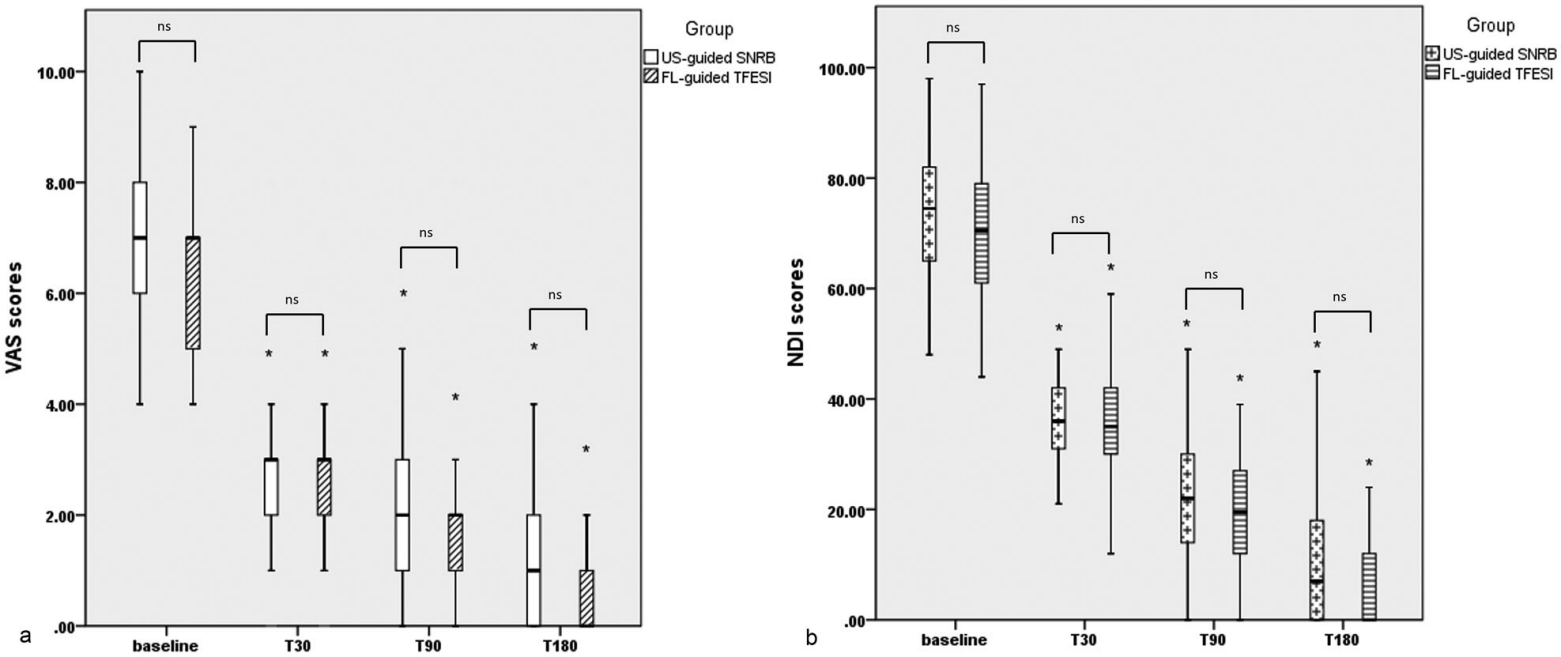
Box plots showing changes in (a) NRS scores and (b) NDI scores in two groups before and after interventions for cervical radiculopathy. ns: not significant; *multiple comparison showing *p* < .001. NRS: Numeric Rating Scales; NDI: neck disability index; US: ultrasound; FL: fluoroscopy; T30: 1 month; T90: 3 months; T180: 6 months after the intervention.
